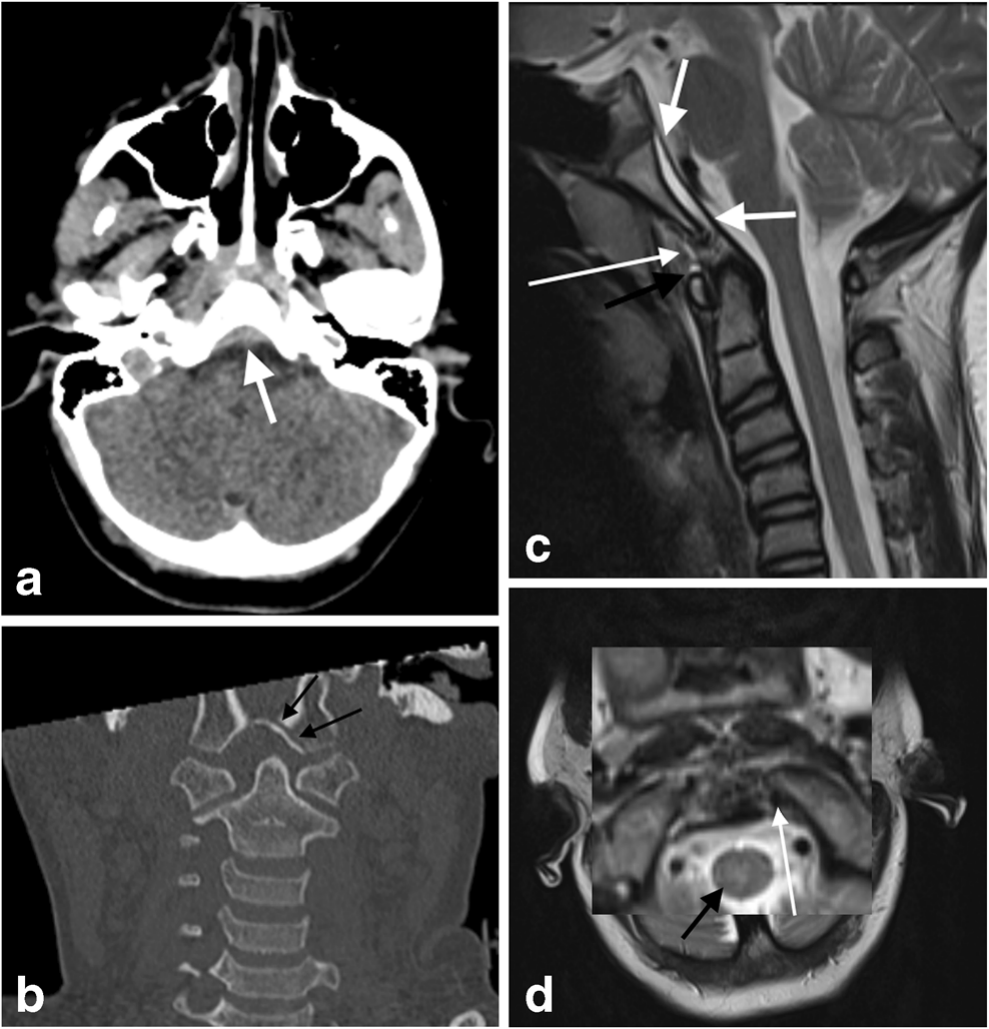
Retroclival epidural haematoma in a 12-year-old female who was a pedestrian struck by a car travelling at high speed: a axial non-contrast CT brain image through the posterior fossa demonstrates hyperdense retroclival abnormality (*white arrow*) in keeping with epidural haematoma; b: coronal reformatted CT image of the craniocervical junction of the same patient demonstrates a left basiocciput fracture extending to the left occipital condyle (type II occipital condyle fracture pattern; *black arrows*). There is asymmetrical widening of the right lateral atlanto-dental space raising the likelihood of right alar ligament disruption; c: sagittal T2-weighted image of the same patient through the craniocervical junction demonstrates the retroclival epidural haematoma (*short white arrows*) associated with traumatic signal change oedema and haemorrhagic fluid tracking between the deep and superficial layers of the anterior atlanto-occipital membrane (*thin long white arrow*) and around the membrane over the superior aspect of the anterior arch of the C1 vertebra (atlas; *black arrow*); d: axial T2-weighted image through the craniocervical junction at the level of the occipital condyles of the same patient with magnified inset demonstrating an intact left alar ligament (*white arrow*) but loss of integrity of the right alar ligament in the comparative contralateral location as a result of rupture. A traumatic oedematous contusion signal abnormality is present in the right and central cervico-medullary parenchyma (*black arrow*)
